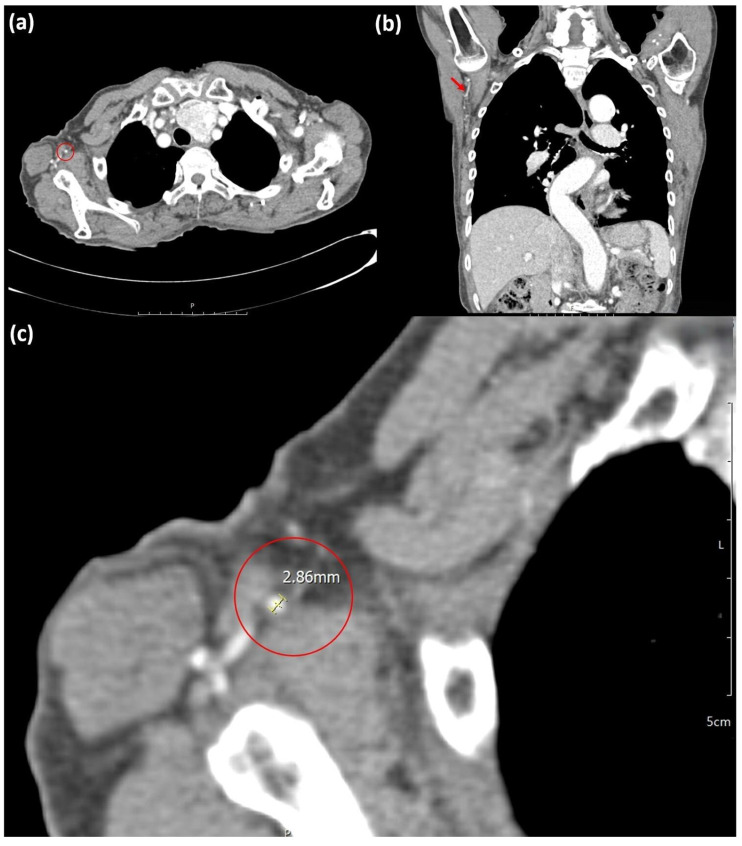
Measurement of the thoracodorsal artery on CT angiography. (a) Axial CT image with the red circle indicating the course of the TDA. (b) Coronal CT image with red arrows indicating the course of the TDA. (c) Magnified axial CT image at a magnification of 491.91, with the red circle indicating the measurement site of the TDA. CT, computed tomography; TDA, thoracodorsal artery.
